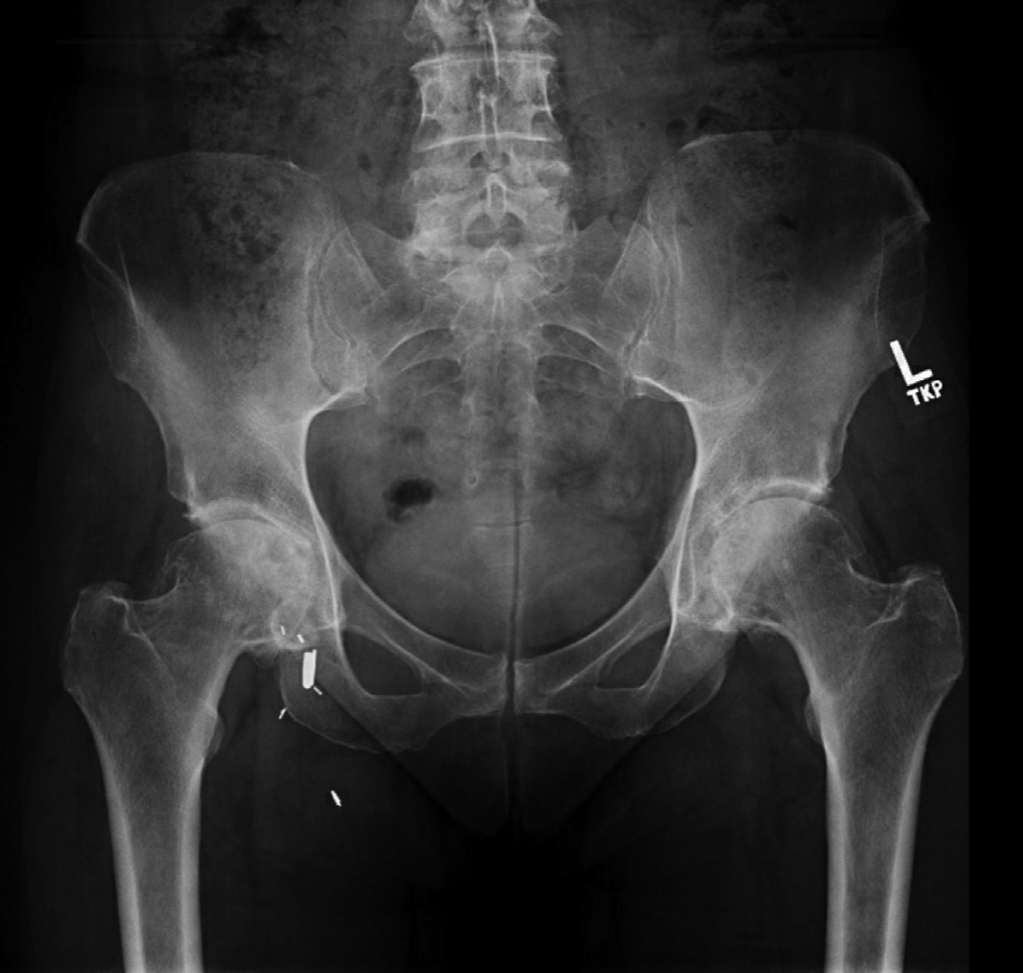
Preoperative pelvic radiograph 2 months after first-stage L-THA, February 2010. Note: Metallic objects seen in the right groin are surgical clips from a remote saphenous vein ligation procedure performed in 1988.
